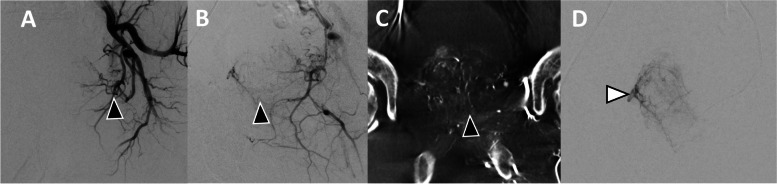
A Left internal iliac artery DSA shows left prostatic artery origin from obturator artery (black arrowhead). B After selective DSA of left prostatic artery it is suspected that both lobes are being supplied by this artery (black arrowhead). C CBCT confirmed perfusion of bilateral lobes of the prostate (black arrowhead). D Subsequently selective contralateral right-side embolization was performed (white arrowhead) followed by embolization of the left prostate gland
